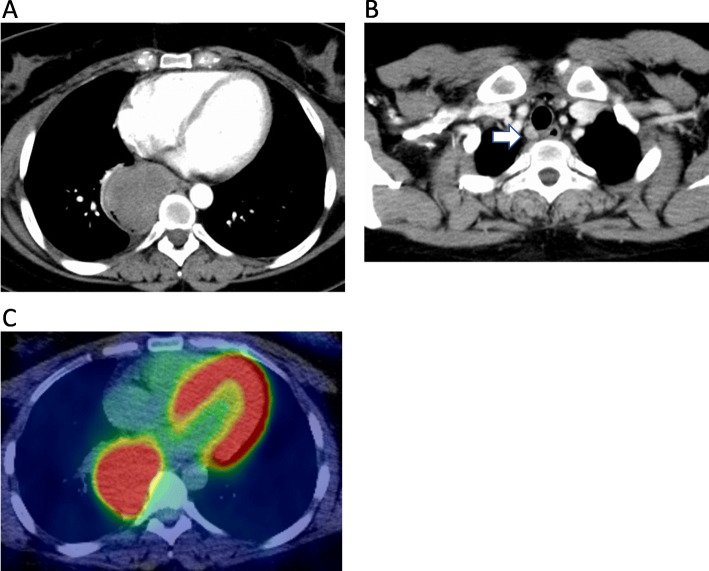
a The CT scan showed a mass of 71 × 61 × 55 mm in the beginning of the lower oesophagus with poor differentiation and progressive contrast enhancement. b The white arrow indicates swelling of up to 12 mm of the right recurrent nerve lymph node on the CT scan. c On FDG-PET, the tumour showed an SUVmax of 11.05, and no abnormal accumulation was found in lymph nodes or other organs. FDG-PET, 18F-fluorodeoxyglucose positron emission tomography
